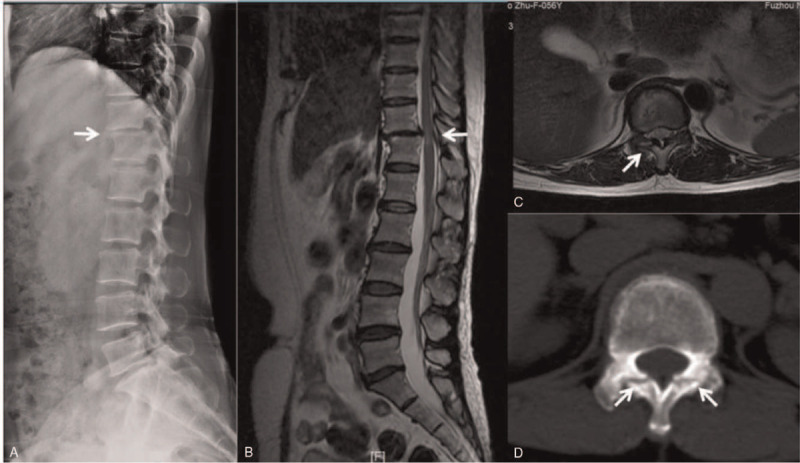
The imaging examinations at the time of presentation. A Sagittal X-ray (A) shows grade I anterior spondylolisthesis, a sagittal T2-weighted image (B) shows severe cord compression, an axial T2-weighted image (C) shows right facet joint hyperintense signal suggesting joint effusion, and an axial CT (D) show bilateral facet joint osteoarthritis at T11/12.
